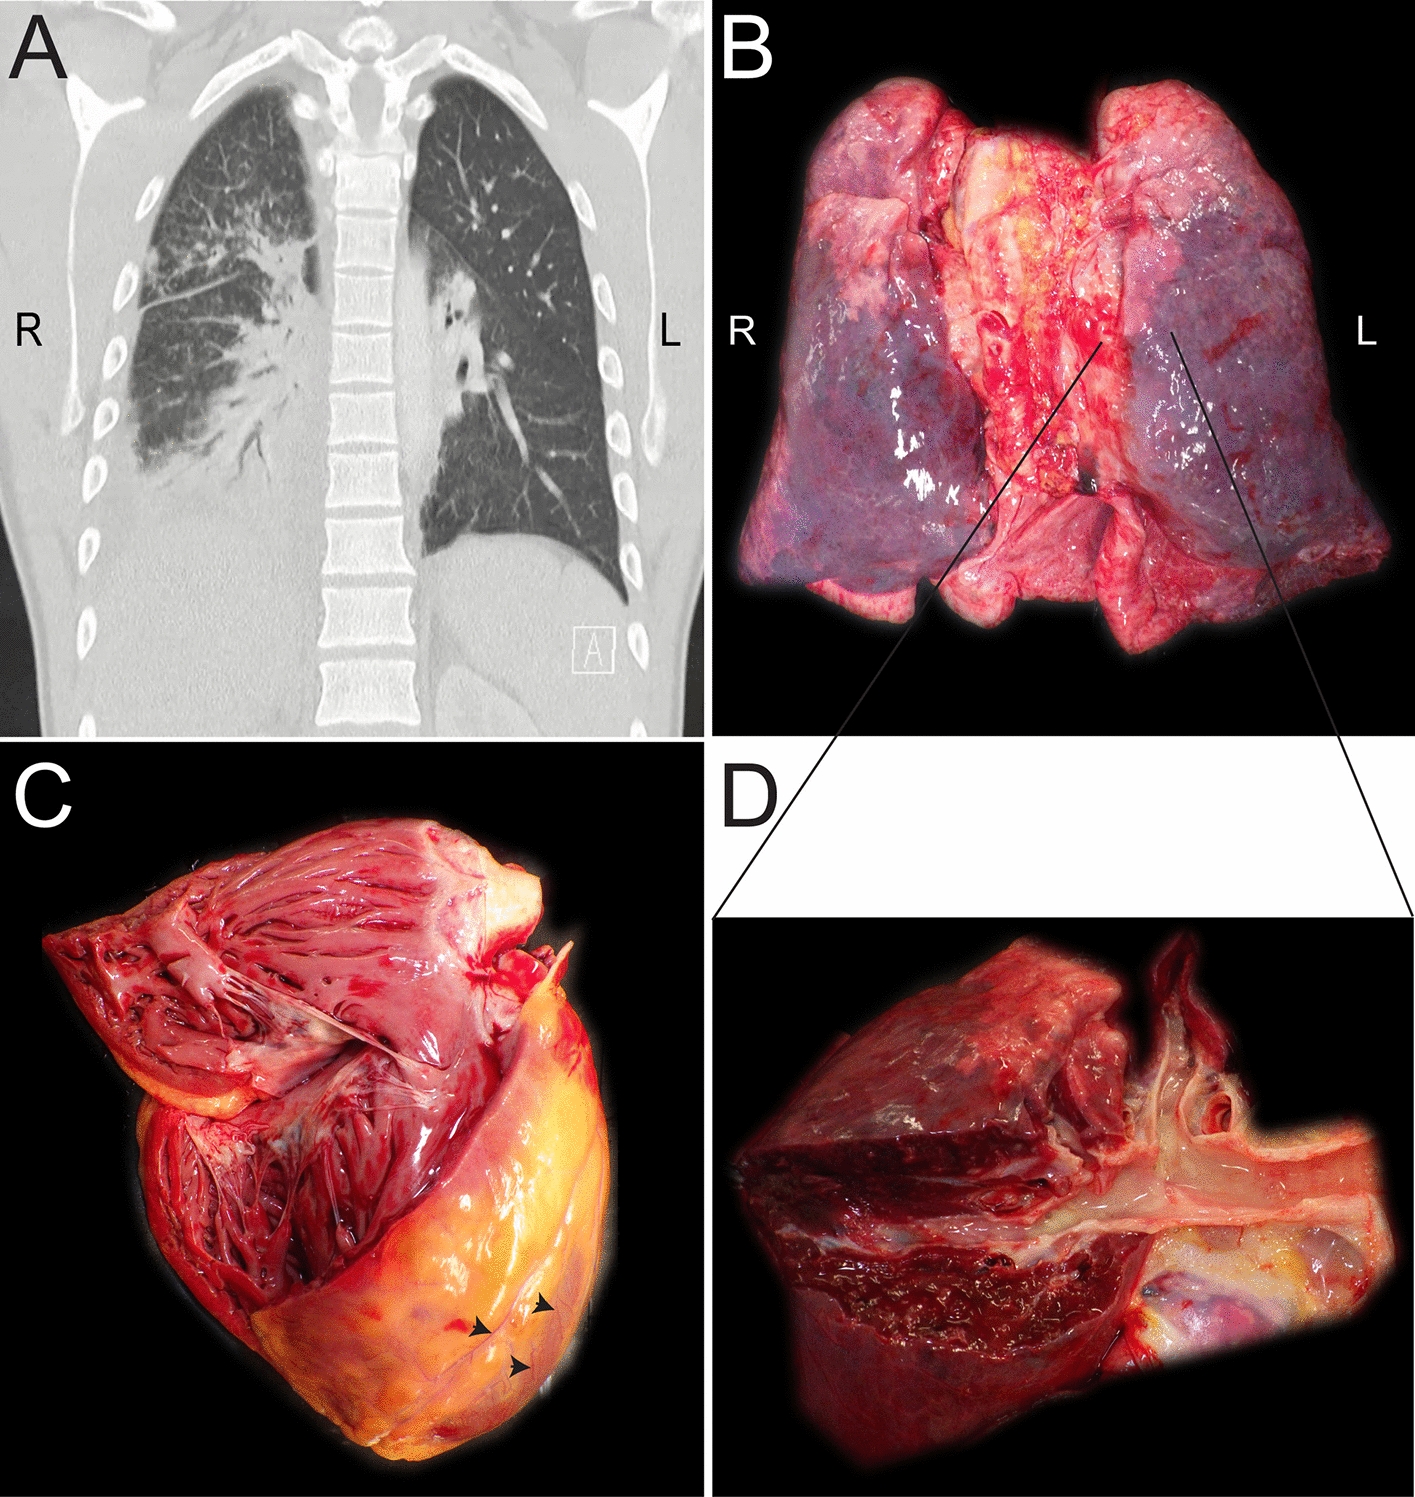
Images of chest computer tomography and gross examination of lung and heart. A. The representative image of the chest CT showed pneumonia with bilateral partially atelectatic lower lobes and peribronchial infiltration. B. Shiny and smooth surfaces of both lungs were demonstrated in the gross examination's representative image. C. The representative image of the heart's gross examination showed a reddish-brown hue with subtle grayish marbling, indicative of the myocardial tissue. An observable perivascular infiltration pattern was present around the small pericardial vessels under the visceral pericardium (arrow). D. The lung's longitudinal section exhibited viscous mucus, as well as a trachea and bronchi filled with purulence
